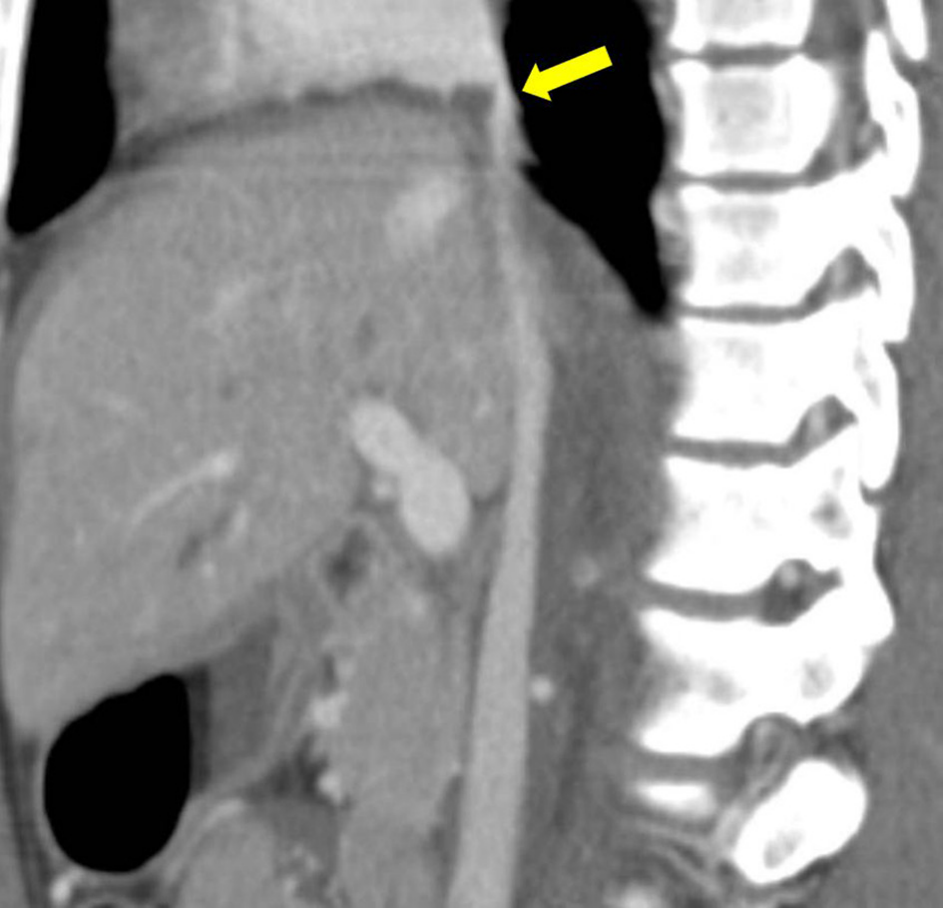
Contrast-enhanced CT abdomen sagittal reformat demonstrates the inferior vena cava thrombus (yellow arrow).
